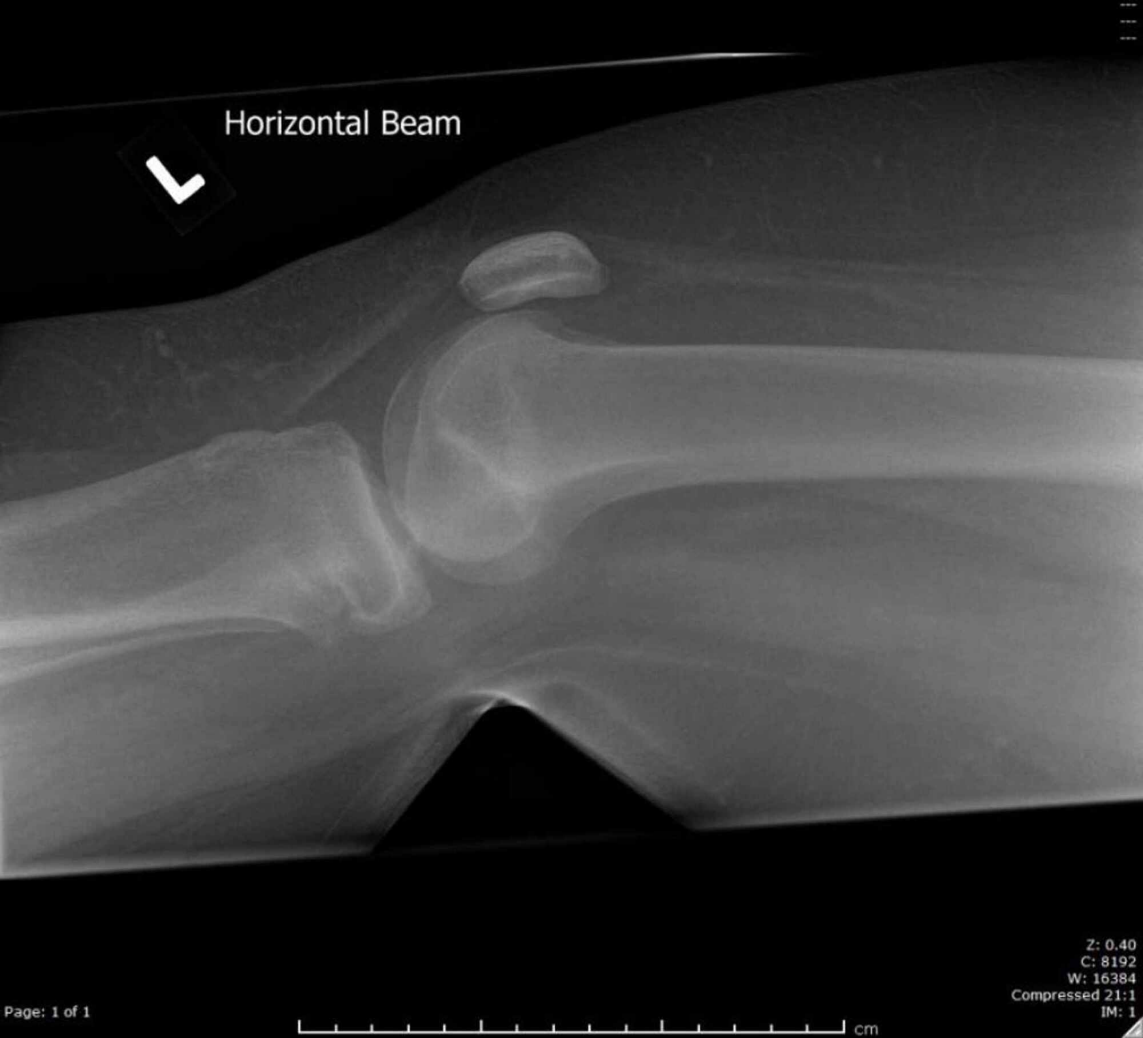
Radiograph of left knee at 12 weeks post injury (lateral view)
